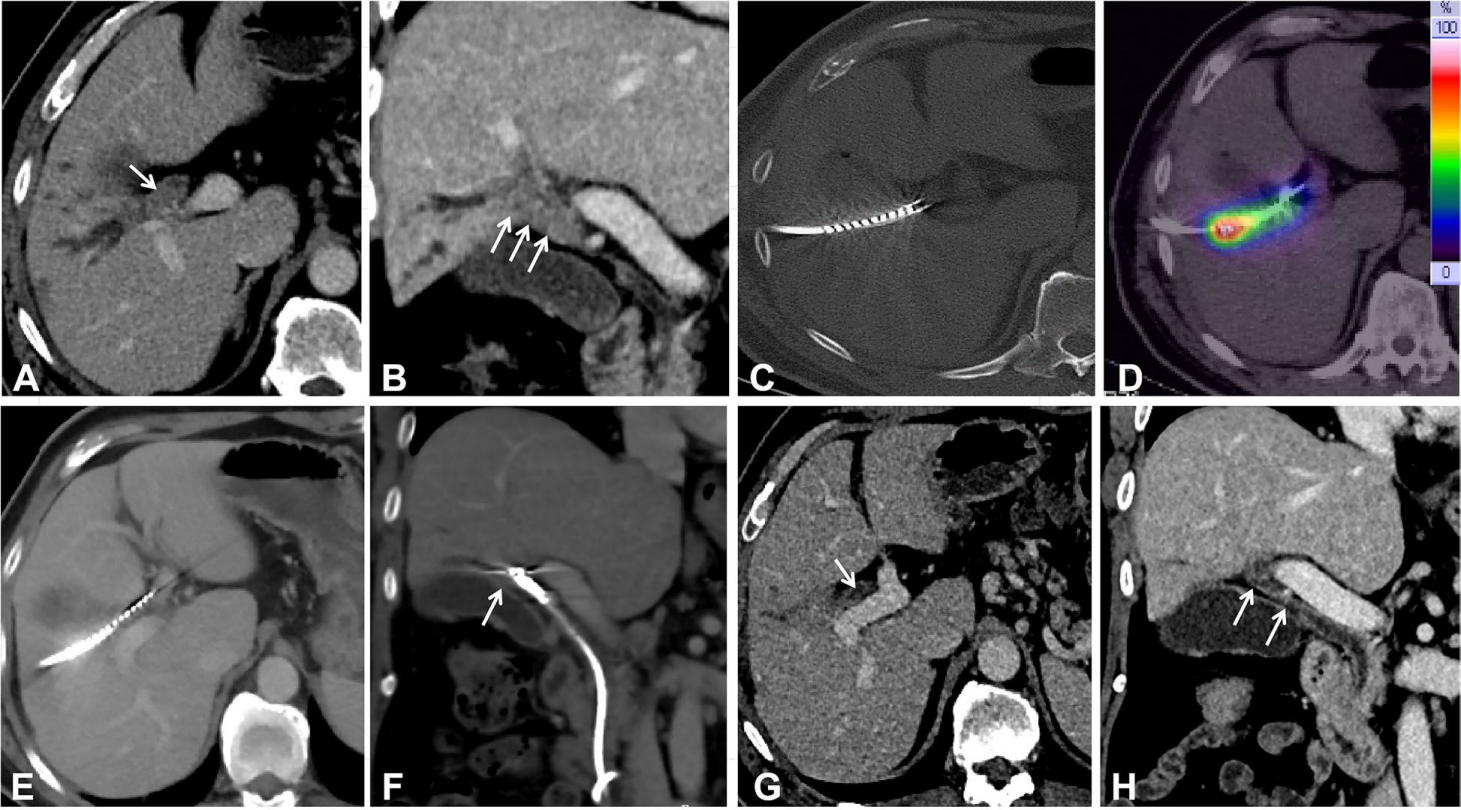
Woman, 55-year-old, pretreatment enhanced CT showed HCCA in the transverse (A) and coronal positions (A) (arrow); posttreatment CT showed that ISS was distributed along the biliary obstruction (C); γ-ray covered HCCA on SPECT with a high local dose and an extremely low dose to the normal surrounding tissues (D); posttreatment enhanced CT showed that HCCA disappeared in the transverse (E) and coronal positions (F) (arrow), and ISS could be successfully pulled out without biliary stenting. After RISB, the patient completed 4 courses of GP chemotherapy, and the local tumor was evaluated as a complete response after 1 year on enhanced CT (G, H)
